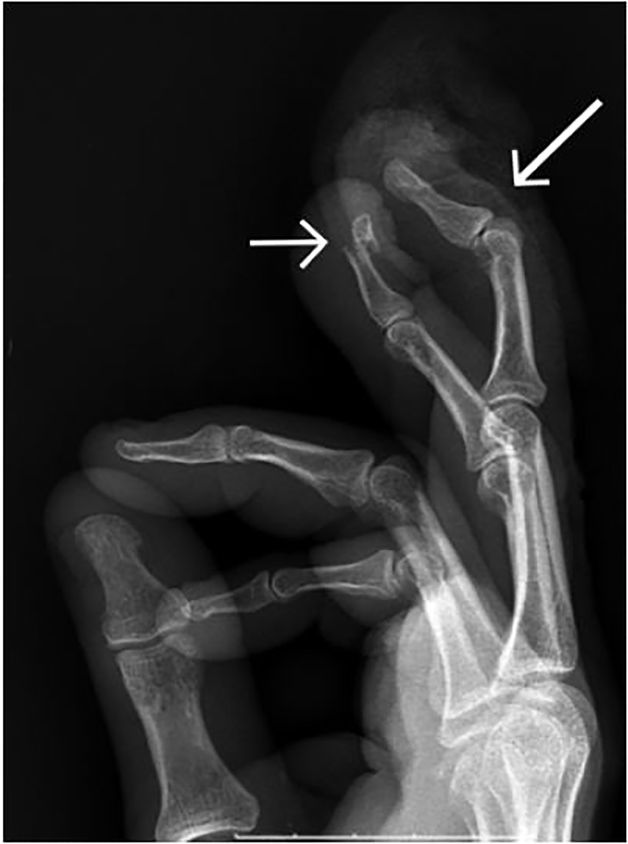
Pre-repair radiograph demonstrating a fracture (short arrow) to the fourth digit and mallet injury (long arrow) to the third digit.
